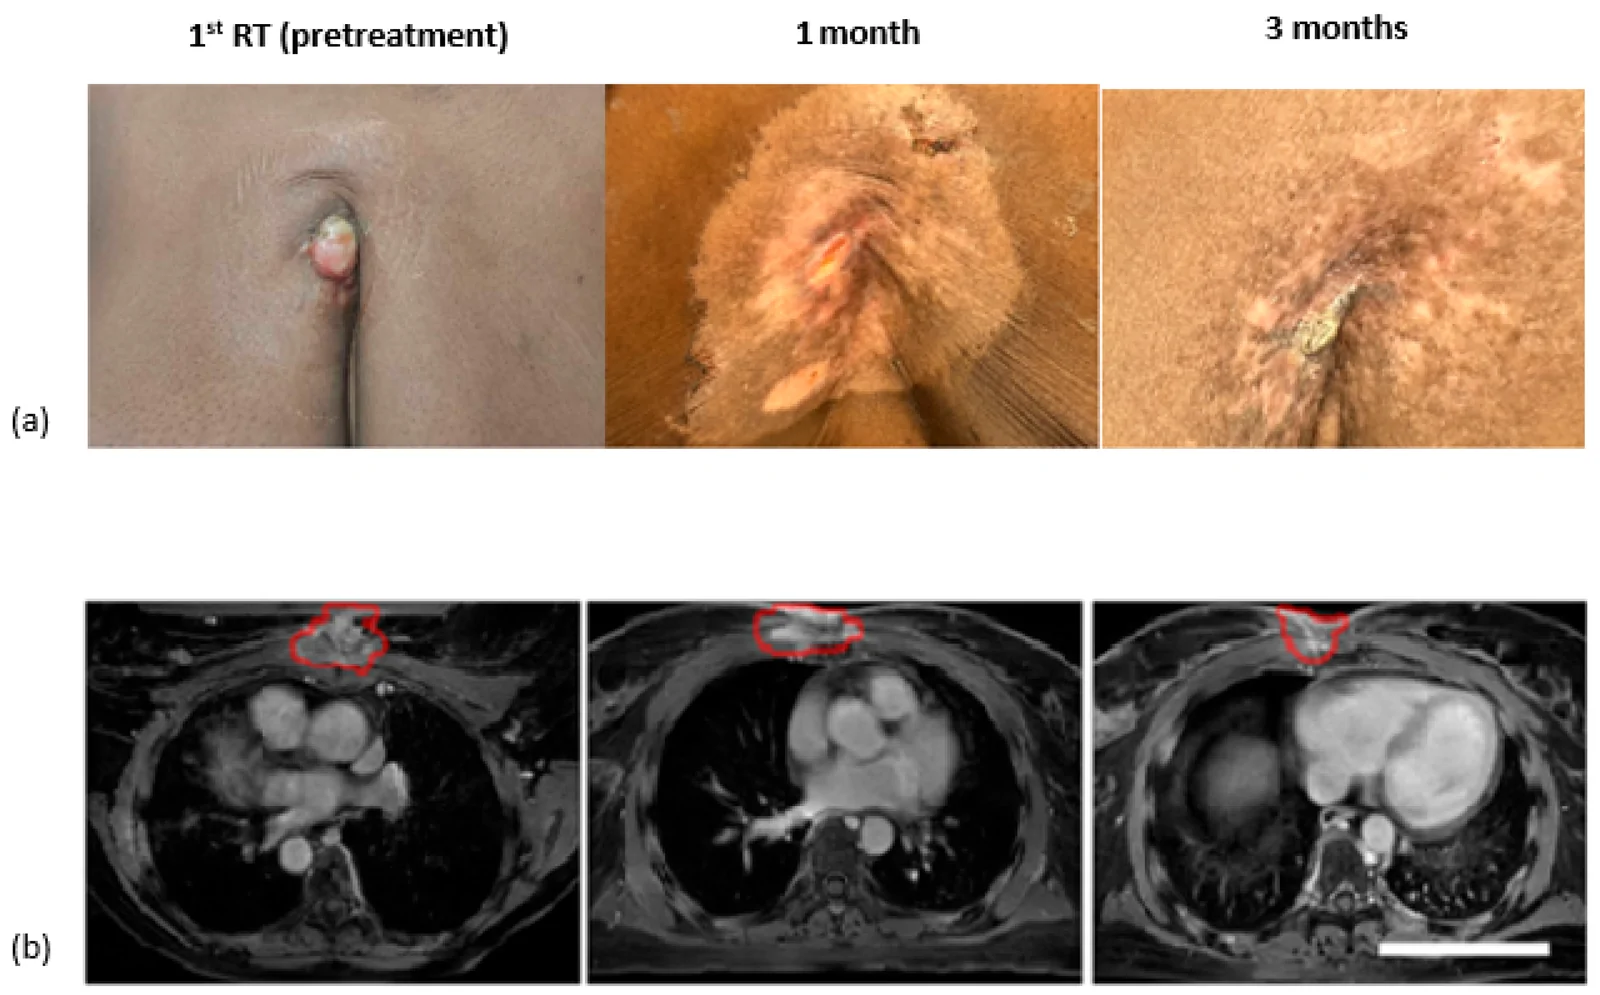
(a) Clinical images of the target lesion for a patient on day 1 of MRgFUS MB treatment and 1 month and 3 months after treatment completion. (b) CE MRI image of the target lesion for the same patient on the 1st day of MRgFUS MB treatment and after 1 month and 3 months of treatment. The red outline in image (b) indicates the ROI contour of the treated tumour. The length bar represents 10 cm.
